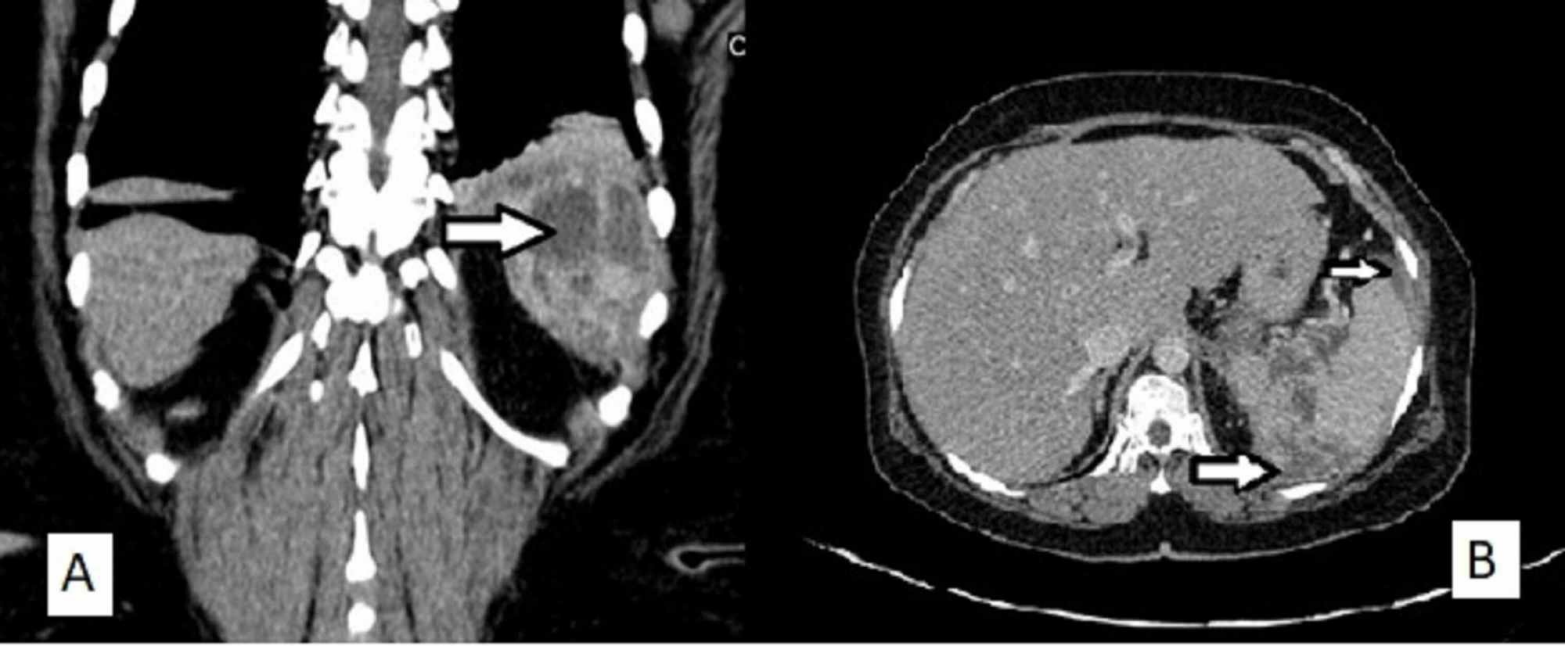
Contrast-enhanced computed tomography of abdomen. (A) Coronal section showing multiple abscesses in the spleen (arrow). (B) Transverse section showing multiple splenic abscesses and perisplenic collection (arrow).
